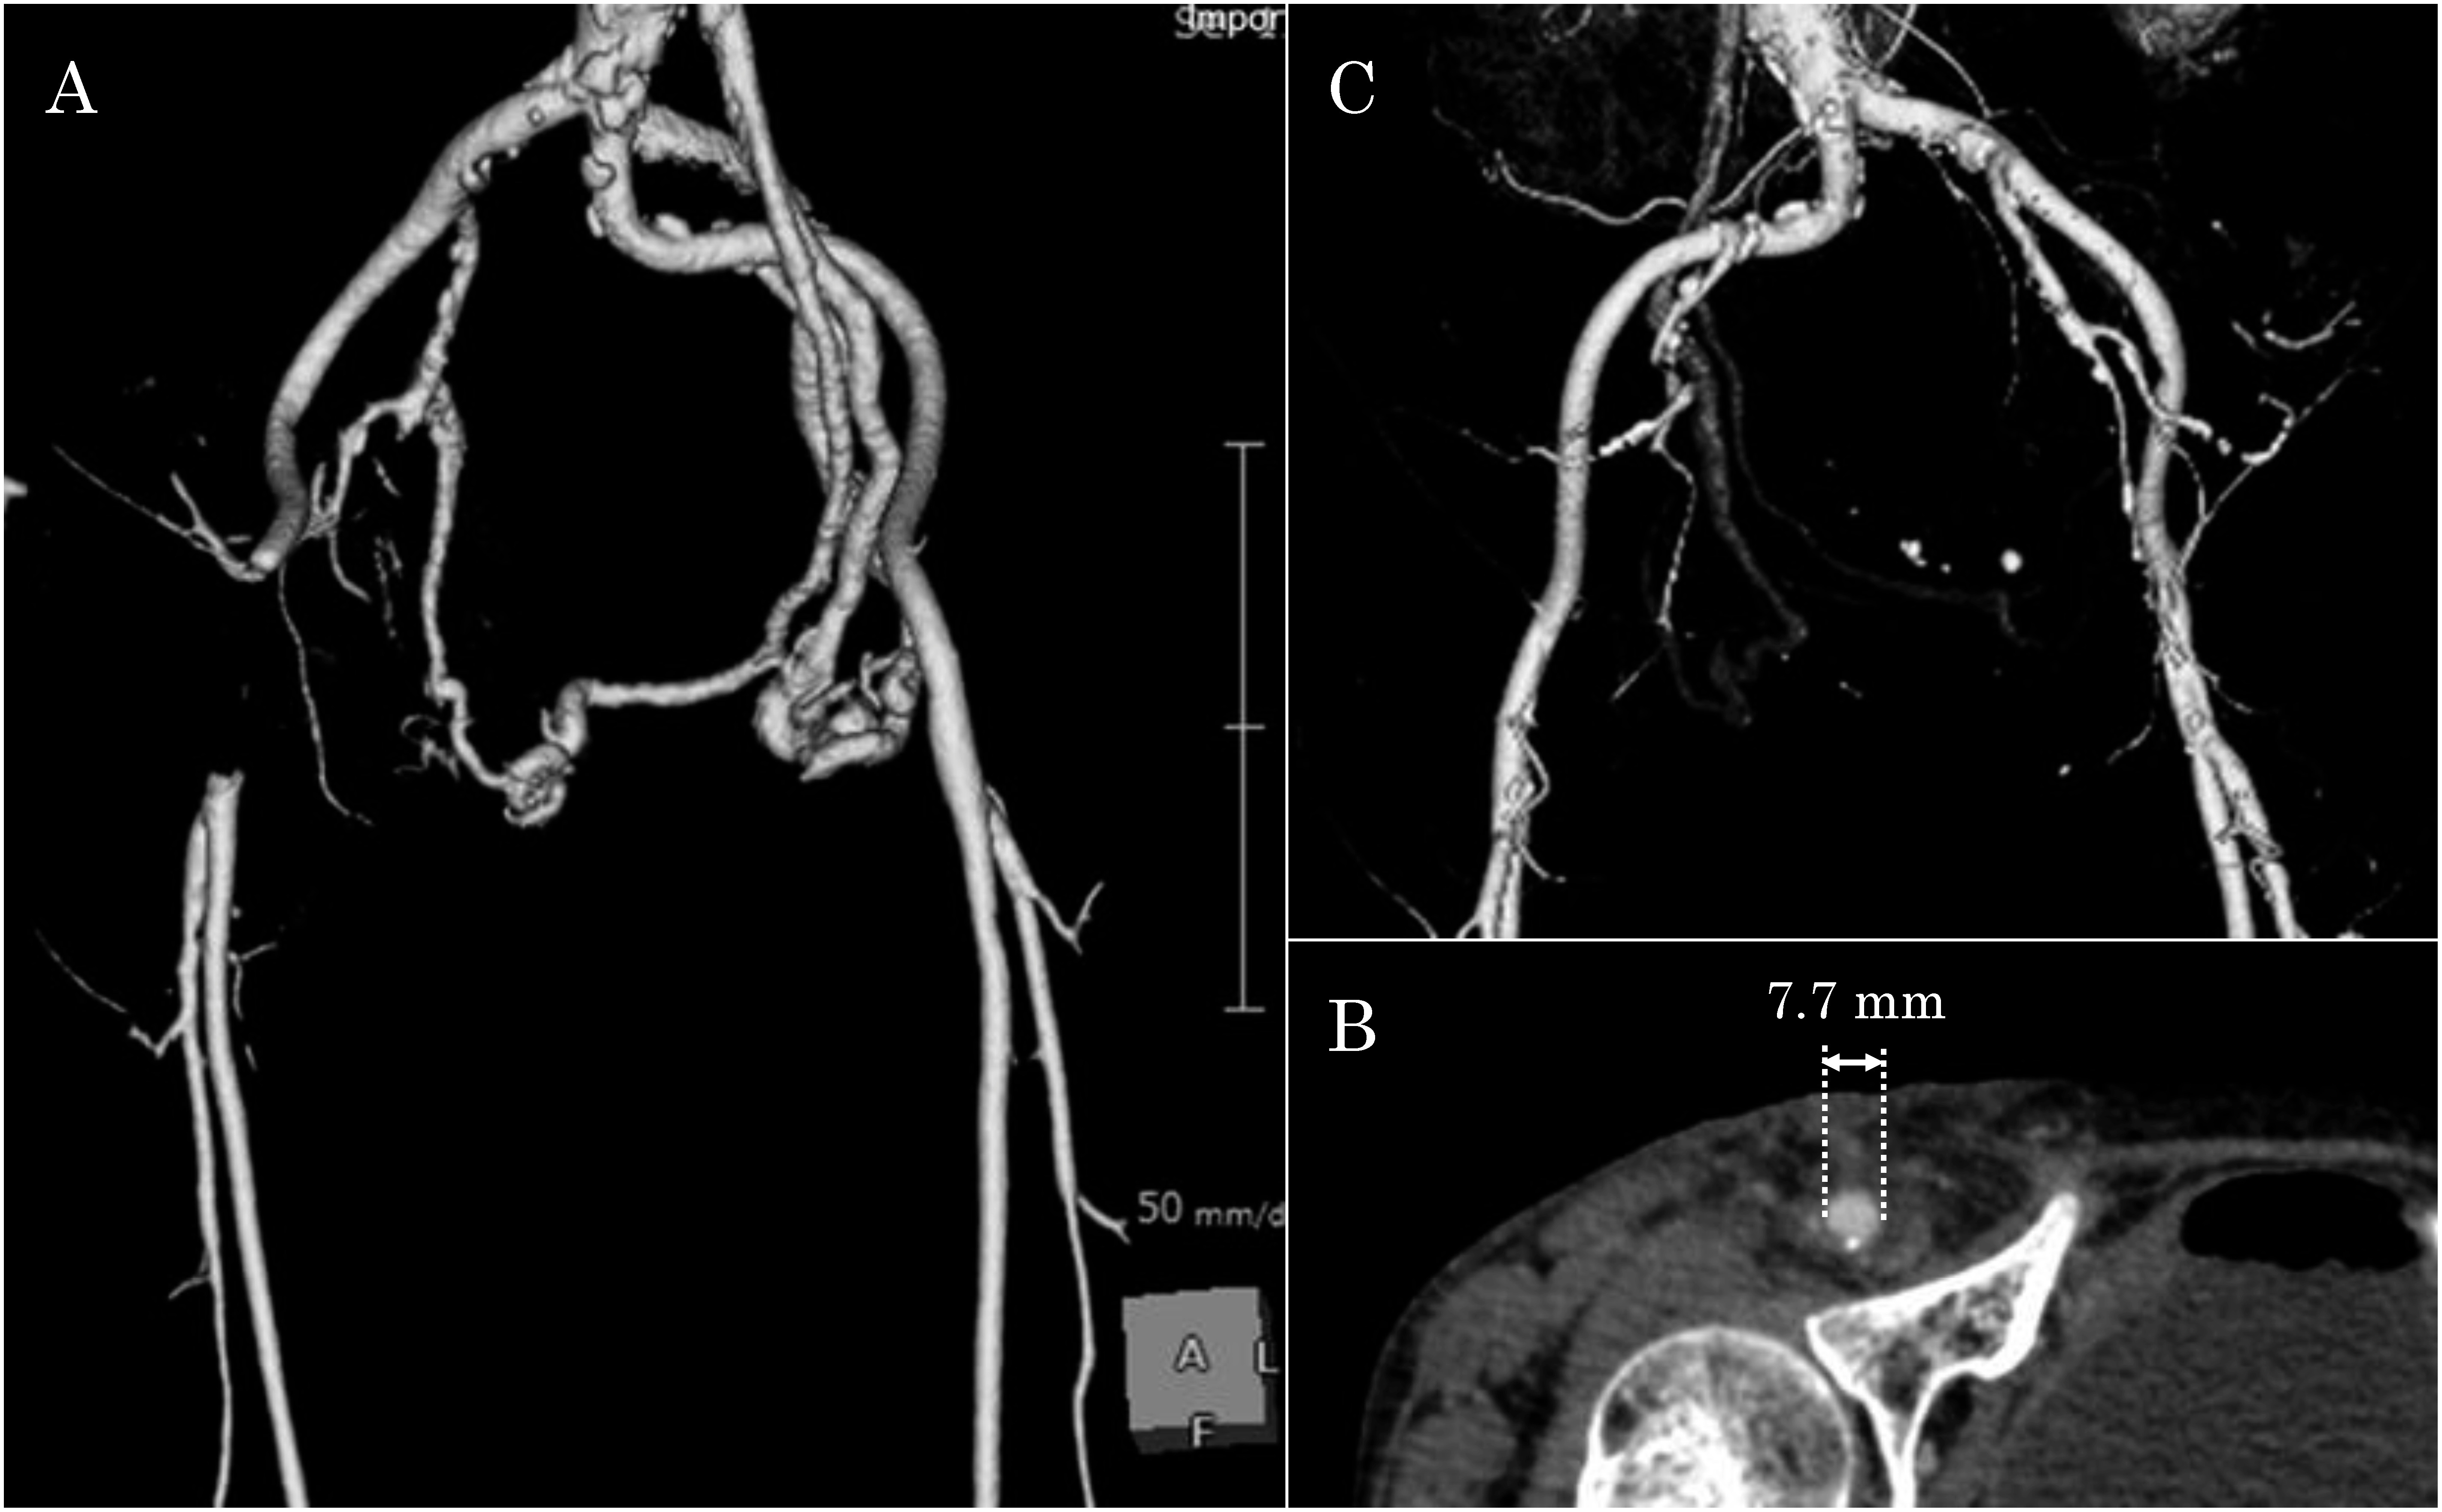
Fig. 1 Contrast-enhanced computed tomography (CT) images. (A) and (B) are the preoperative 3-dimensional (3D)CT and axial CT images. (A) shows the occlusion of the right common femoral artery (CFA) and thrombus from the right external iliac artery to the CFA. (B) demonstrates that the diameter of the CFA is 7.7 mm. (C) is the postoperative 3DCT and shows that the anastomosis has no stenosis.
